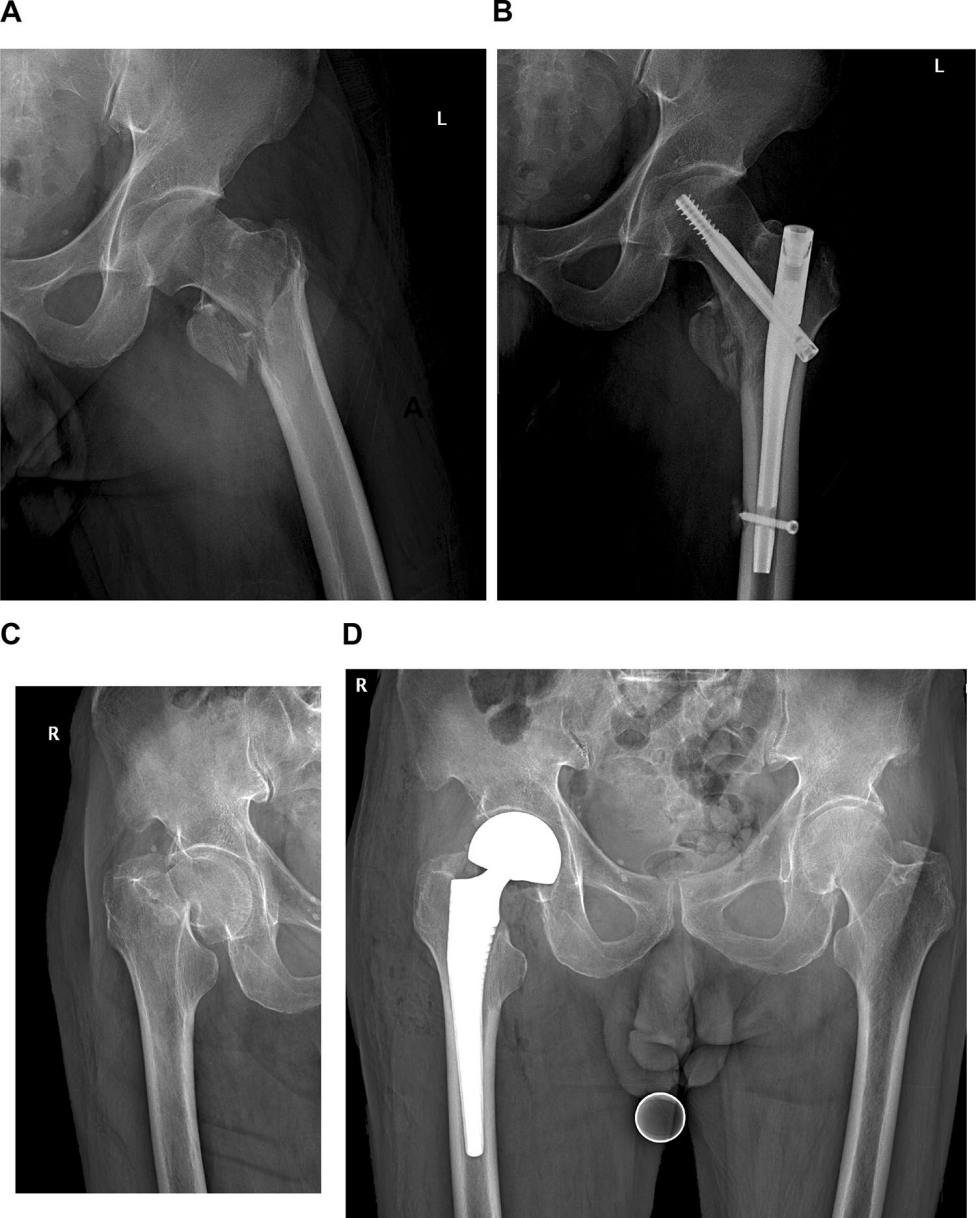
The radiographs of two major ways of fracture fixation in cases. X-ray images of a patient with intertrochanteric fracture before (A) and after RIF (B). X-ray images of a patient with femoral neck fracture before (C) and after HR (D)
